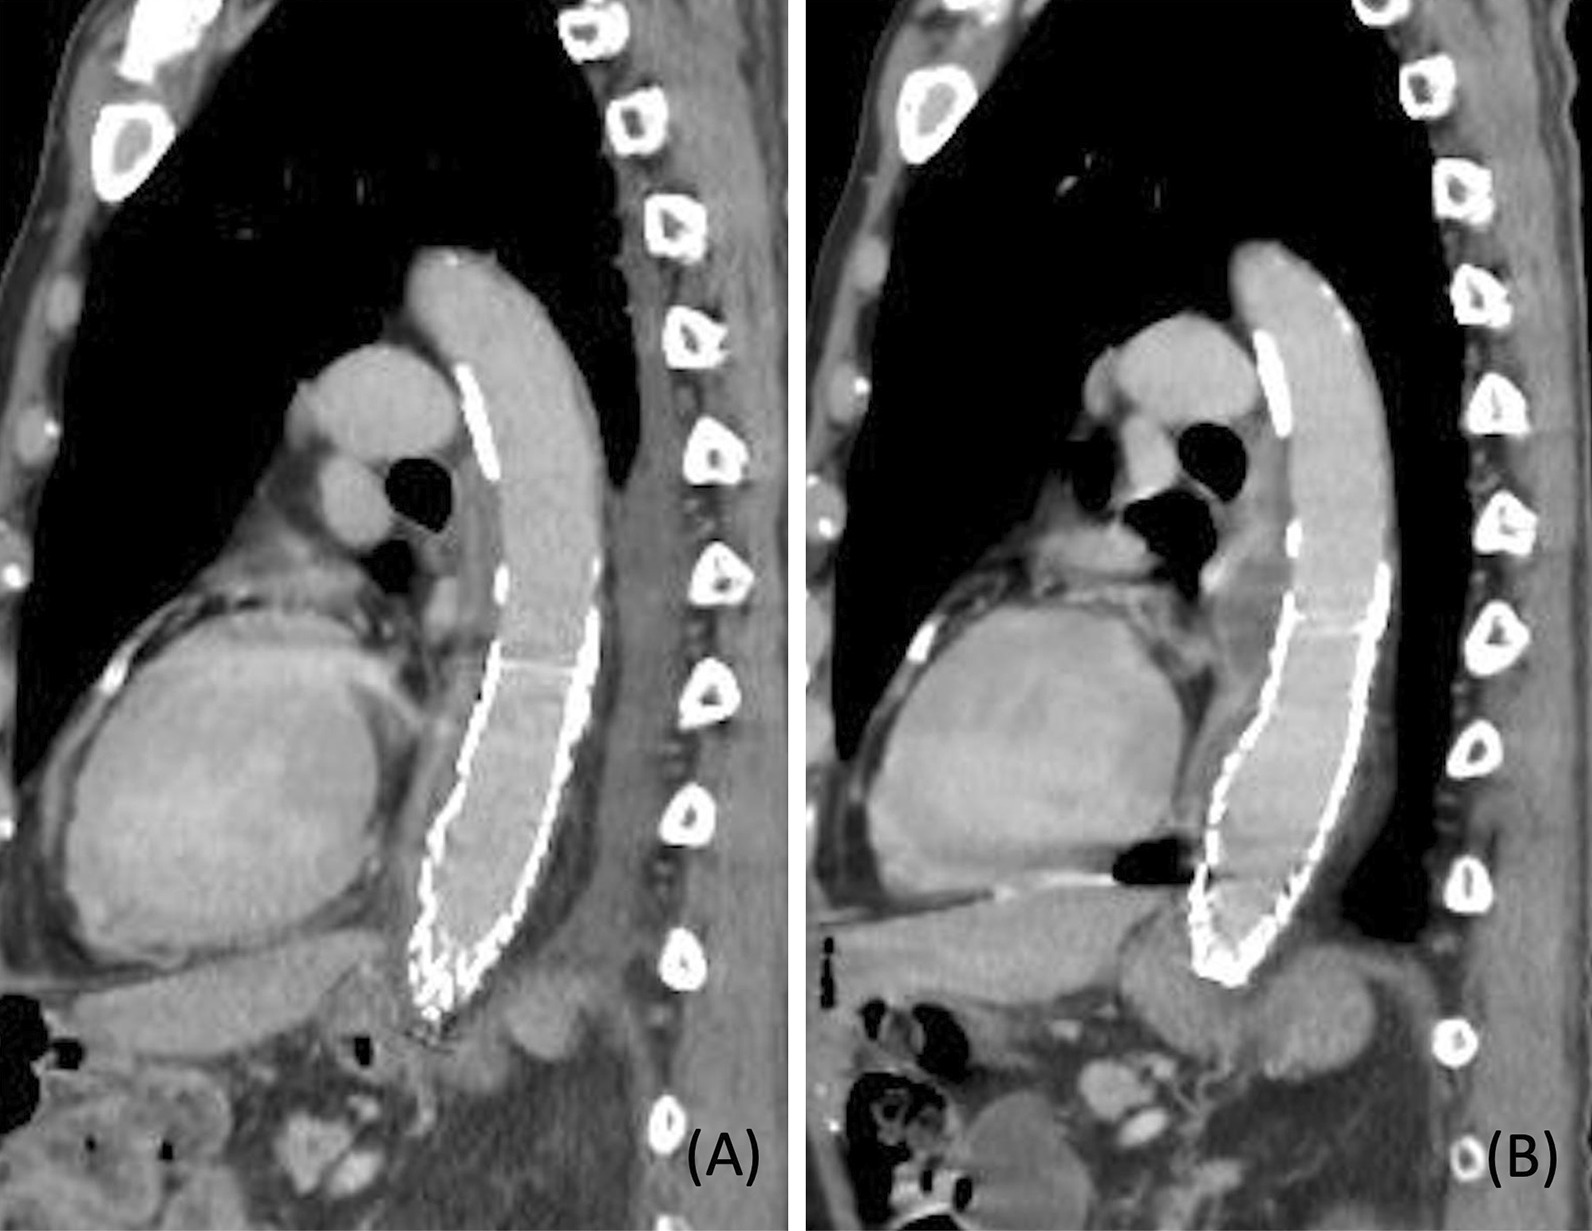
CT performed 17 days after TEVAR revealed that the abscess cavity had extended beyond the proximal edge from the implanted stent graft, i.e., in the area uncovered by the graft (A: three days after the initial TEVAR; B: 17 days after the initial TEVAR)
